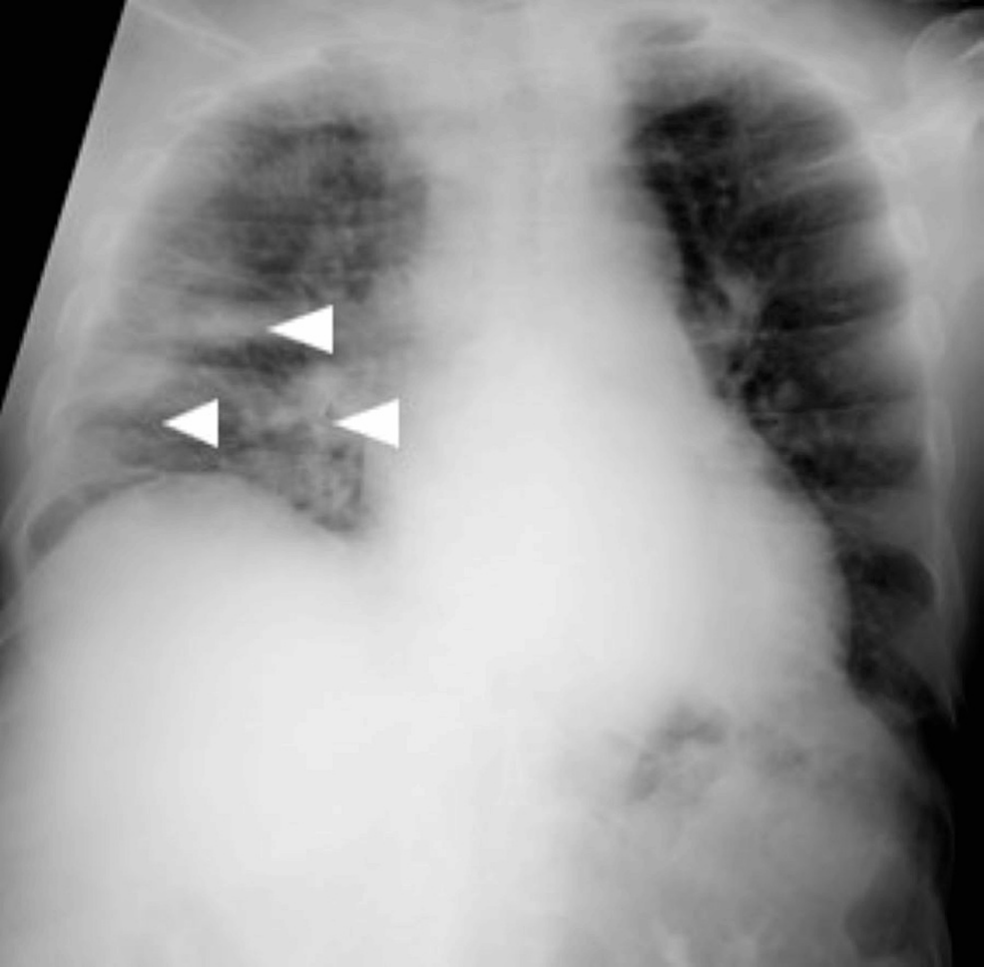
Chest X-ray of patient two The image revealed a right lung volume reduction with patchy and nodular air space opacities in the mid-to-lower zones (arrowheads) and borderline cardiomegaly with a cardiothoracic ratio of 0.55.
